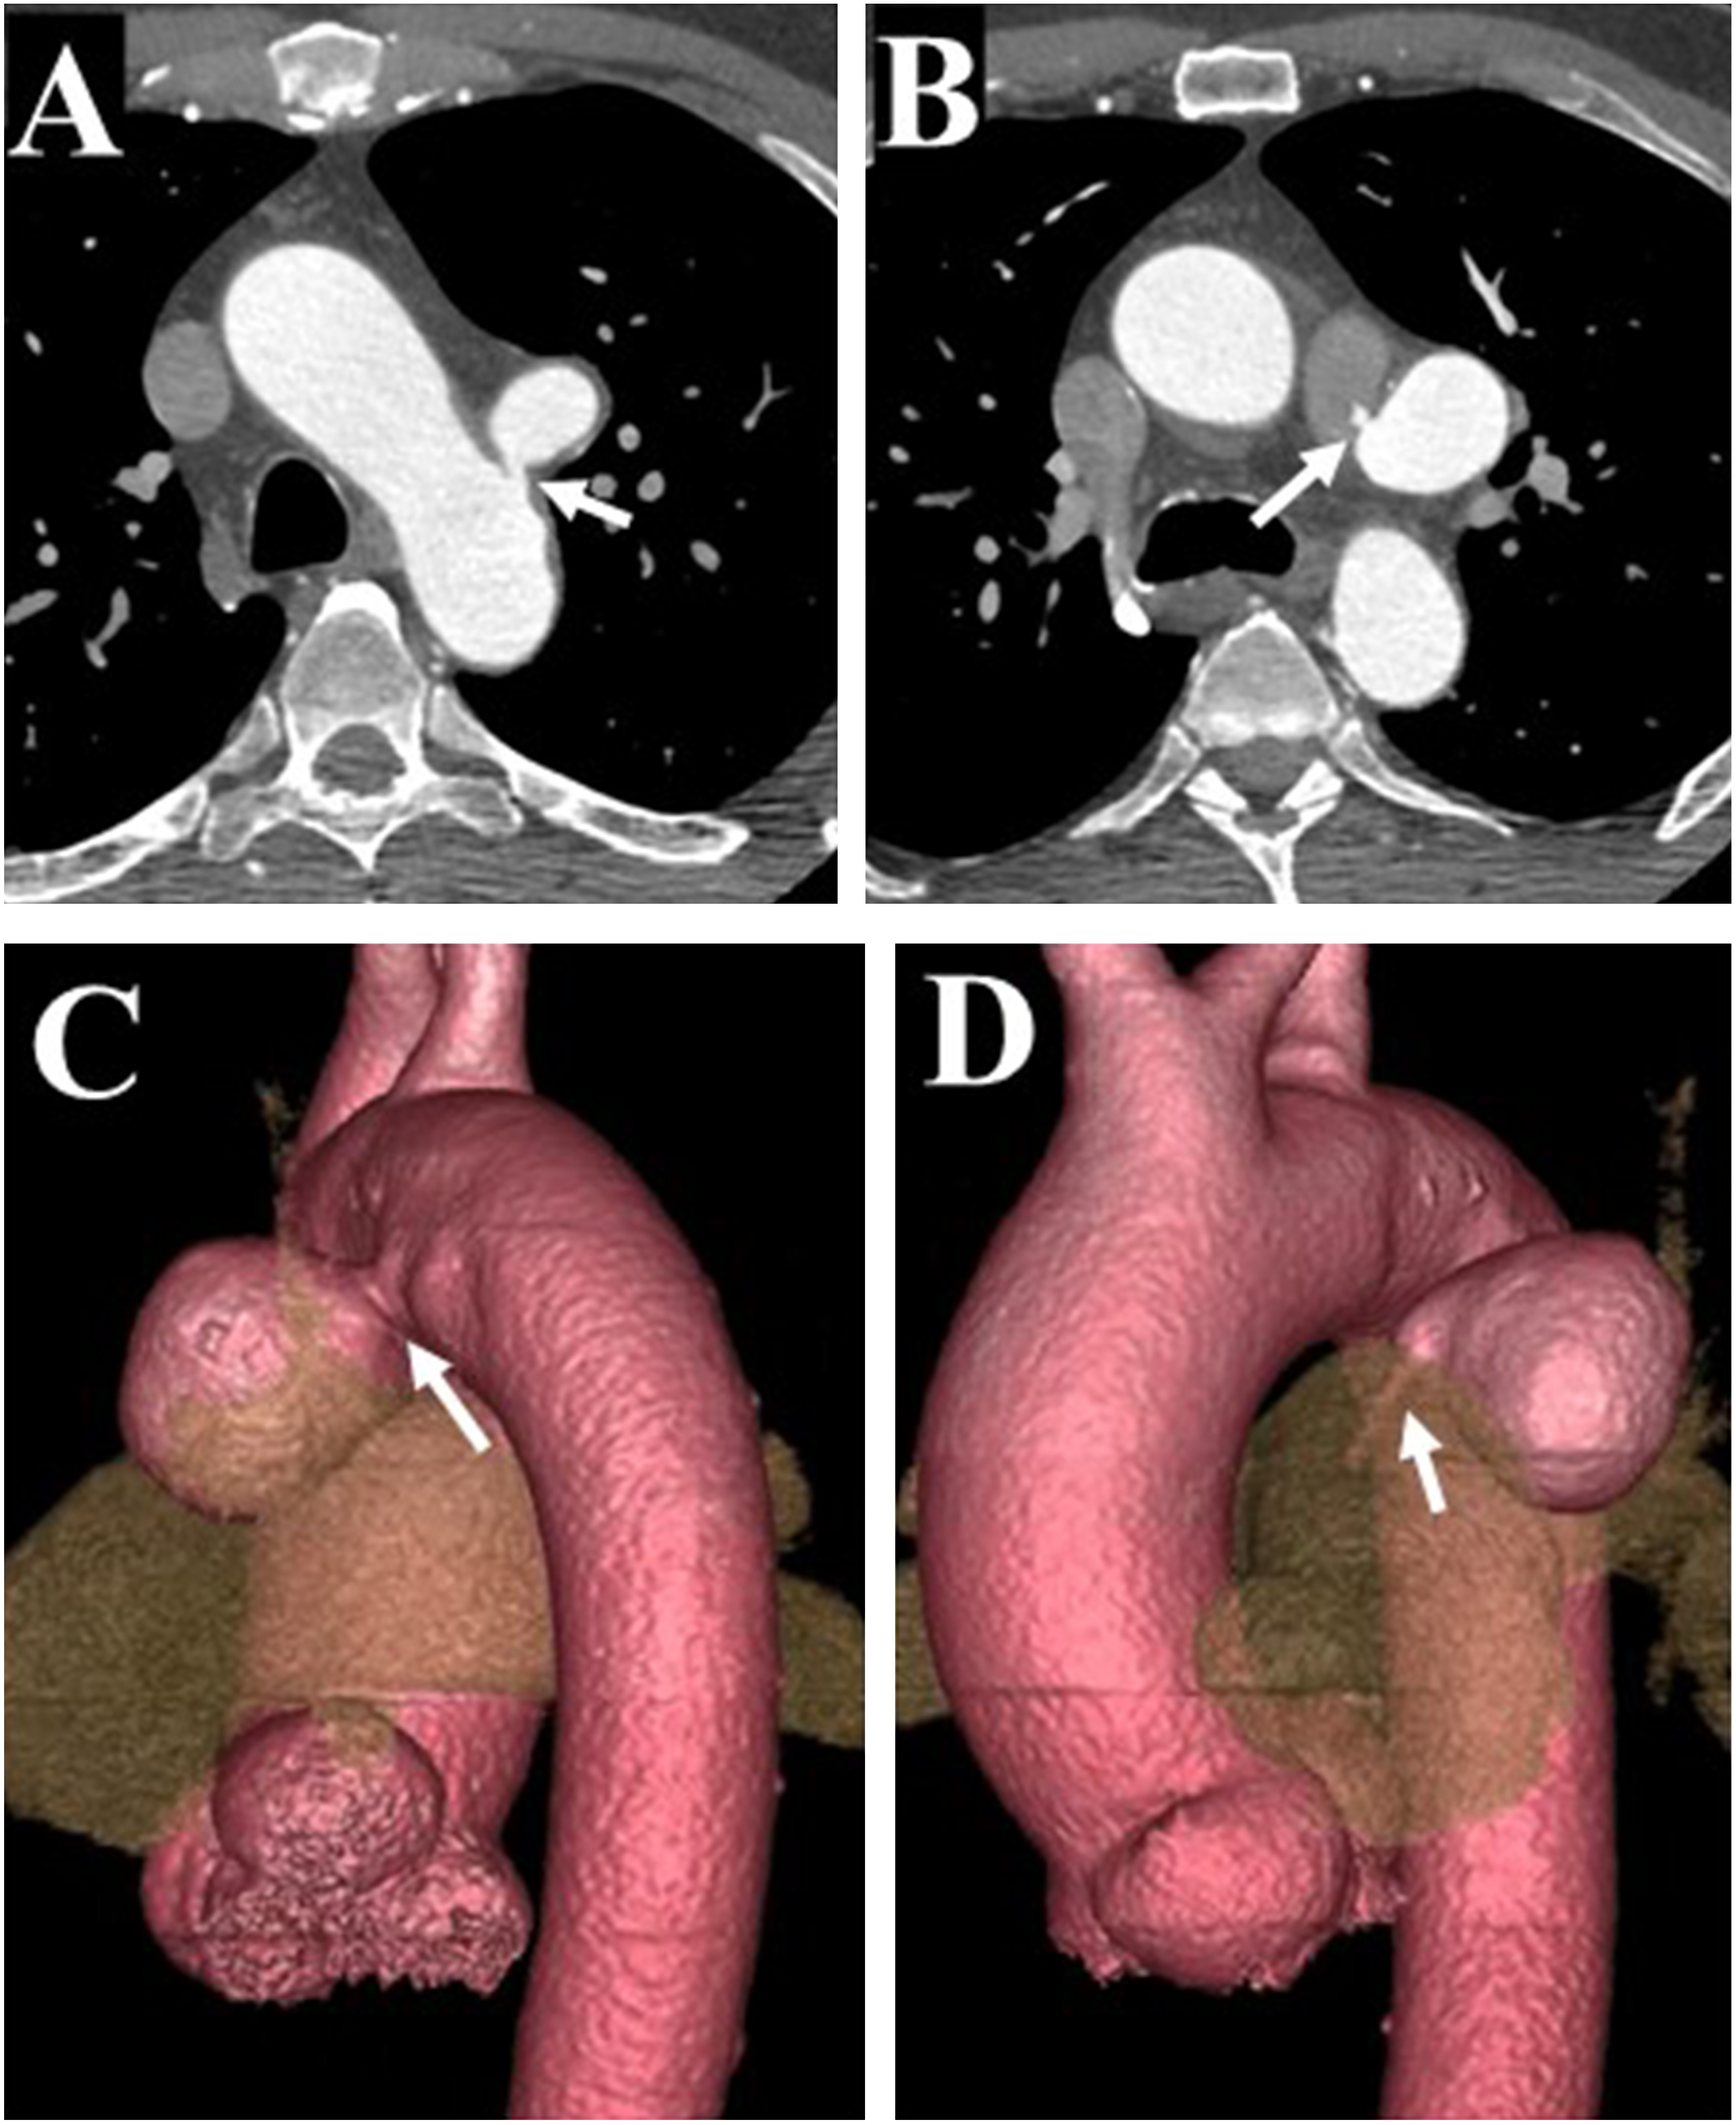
Fig. 1 Preoperative computed tomography. Fistula from the minor curve of the aortic arch to the aneurysm is confirmed (A, C; white arrow). Fistula from the aneurysm to the main pulmonary artery is confirmed (B, D; white arrow).
